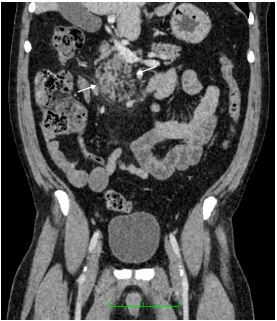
Coronal computed tomography view showing inflammatory stranding of the pancreatic head (arrows).
